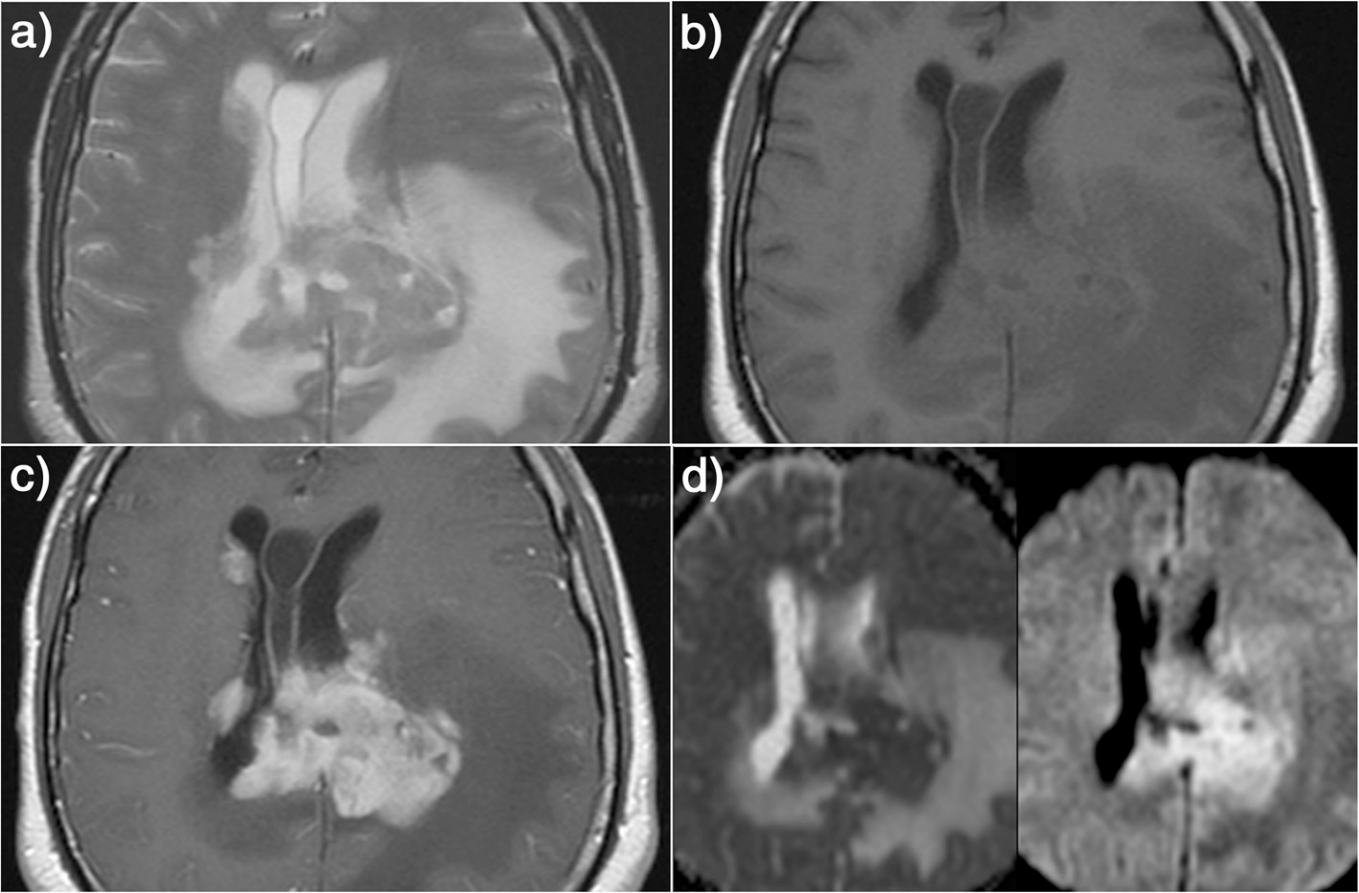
Axial T2WI, T1WI and contrast-enhanced T1WI as well as ADC and DWI maps show the classic appearance of PCNSL where the mass is isointense to mildly hypointense on T2WI and isointense on T1WI (relative to gray-matter) and shows predominantly solid, intense contrast enhancement (1a-1d). The mass is also in a typical distribution abutting a CSF-containing space (in the splenium of the corpus callosum abutting the lateral ventricles in this case). The mass is associated with extensive perilesional T2-hyperintensity. On ADC and DWI, the mass shows diffusion restriction.
